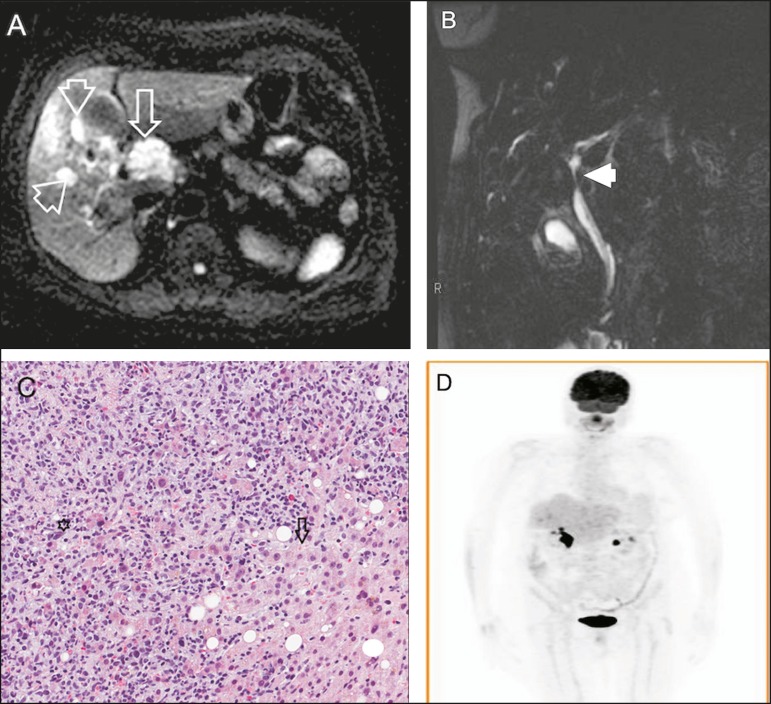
A: Axial diffusion-weighted MRI (b: 600 s/m2) showing a 4-cm
